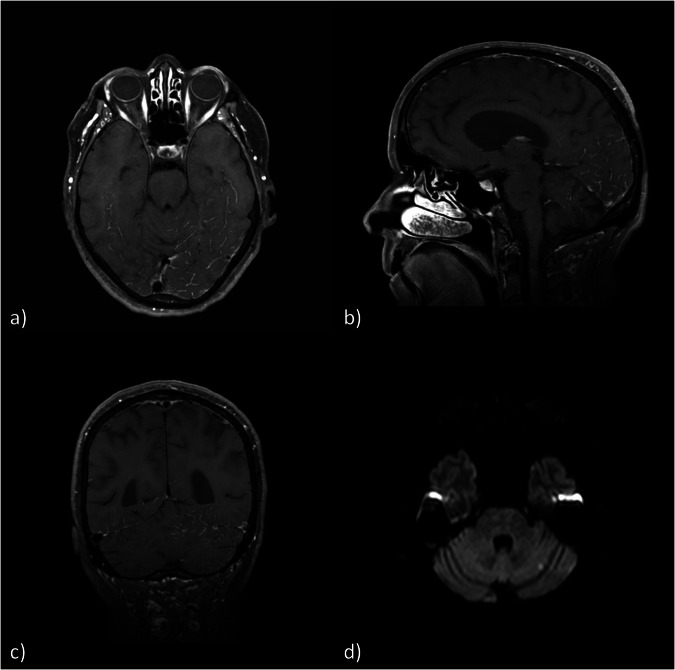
MRI examination 3 h after diagnostic panangiography. This patient was not included in the statistical analysis as the images were acquired from a different MRI device (Philips Achieva 3 T, Best, The Netherlands). a Axial reconstruction of three-dimensional T1-weighted sequence 5 min after contrast administration showing a pial enhancement in the posterior cerebral artery territory; sagittal (b) and coronal (c) reconstructions of the same sequence. d DWI sequence showing the only present microembolisms in the left SUCA territory. DWI, Diffusion-weighted imaging; MRI, Magnetic resonance imaging; SUCA, Superior cerebrallar artery
